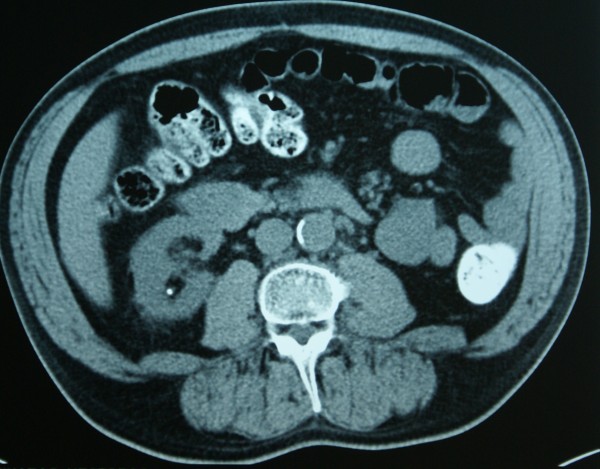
Abdominal computed tomography (CT) scan showing a calcified 2,5 × 2,7 cm mass at the lower pole of the right kidney.
